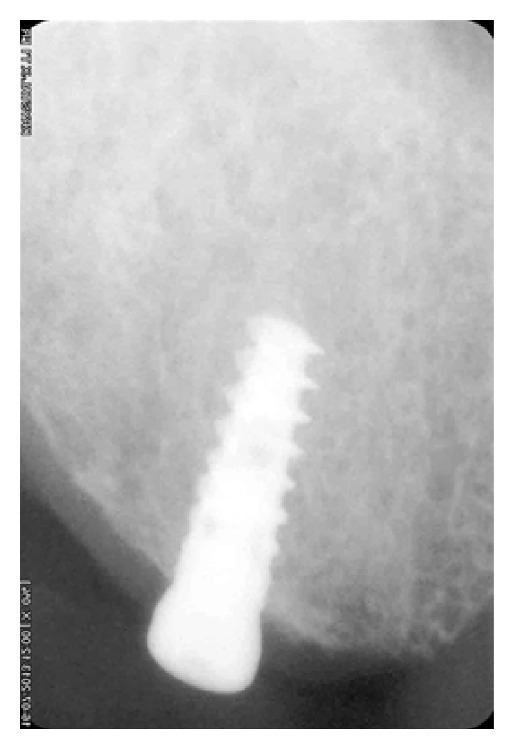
Intraoral periapical radiograph of implant after 4 months of osseointegration.
